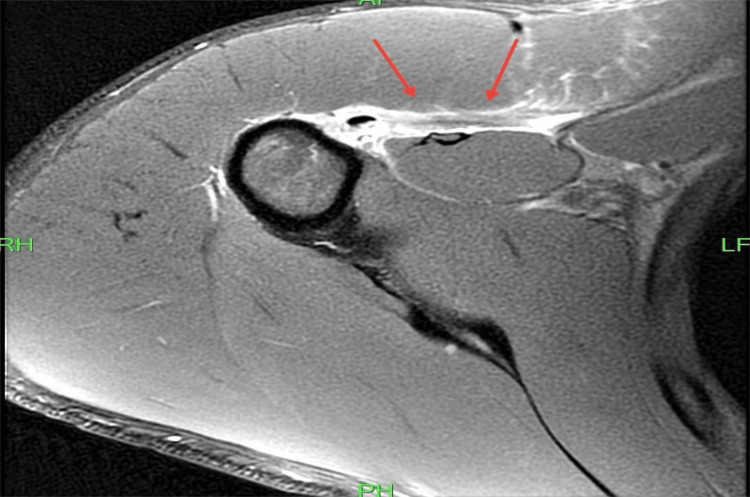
Right shoulder magnetic resonance imaging. Pectoralis major sternal head tendon rupture with fluid collection (red arrow). RH: right head; LF: left foot; PH: posterior head
